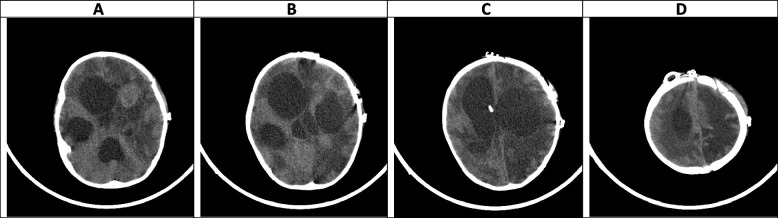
(the Brain CT SCAN without contrast, day 65). A-D All of the findings at the previous two CT scans are again present, including significant hydrocephalus, again suggestive of either EVD malfunction or severity of hydrocephalus. Diffuse hypodense areas seen within the brain parenchyma suggestive of brain edema. The hemorrhage of mentioned largest of left frontotemporal lesions is significantly improved at this CT SCAN (the only remnant of hemorrhage is seen)
